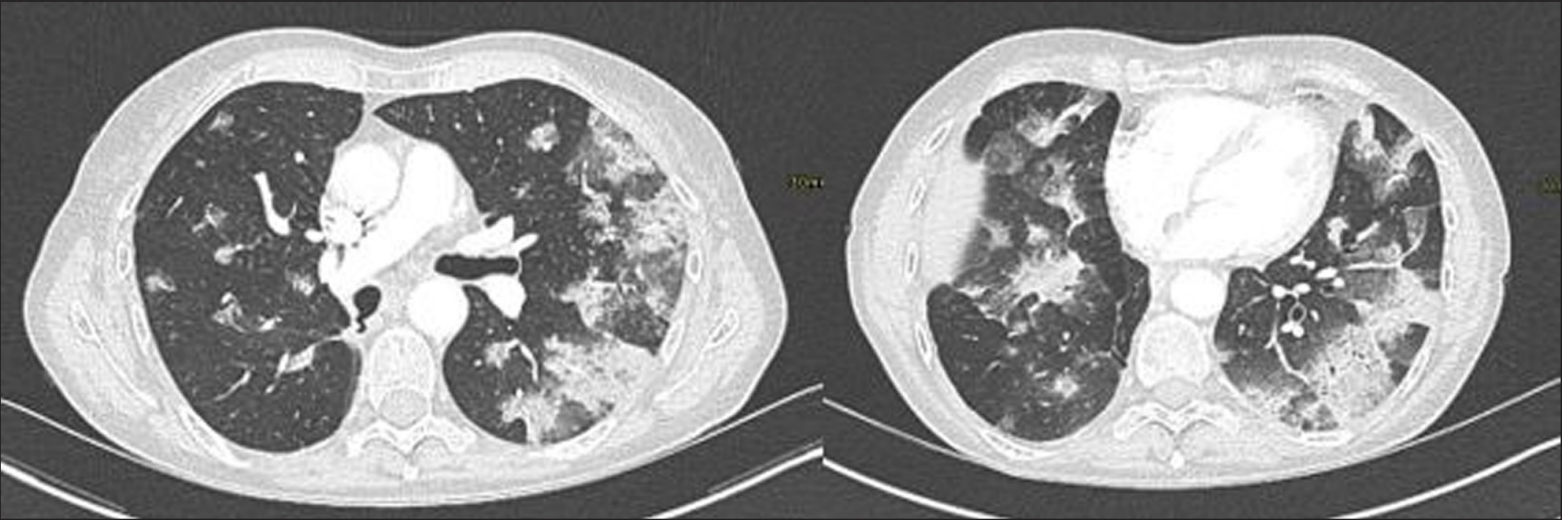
False-positive CO-RADS chest CT for COVID-19 when compared to RT-PCR test but considered true positive by treating clinician: 74-year-old man with four weeks of diarrhoea and two weeks of coughing. CO-RADS score of 5. At the time of scanning, no residual respiratory symptoms or fever were present and RT-PCR test was negative three times.
